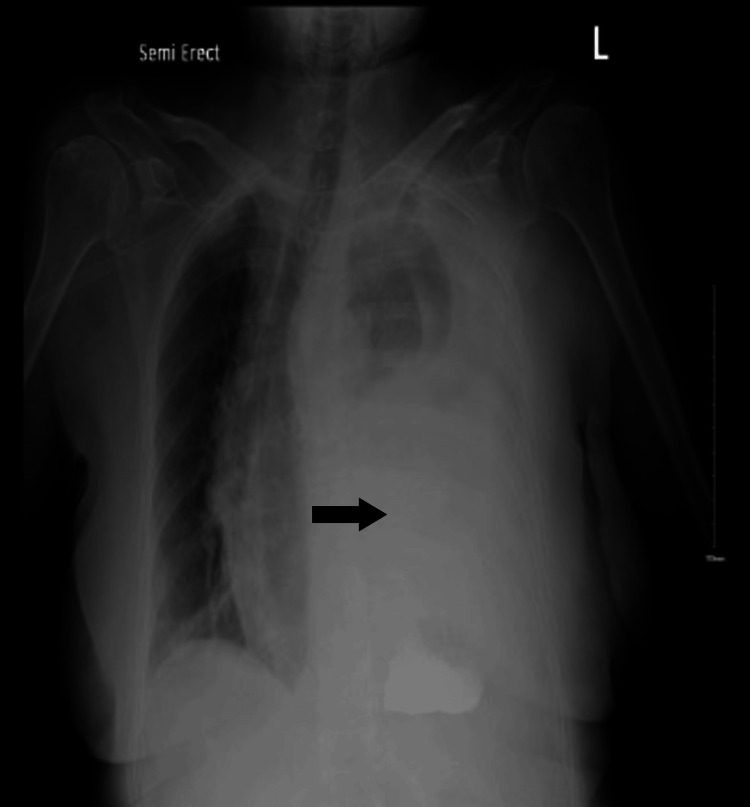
Chest X-ray revealing large left-sided pleural effusion.
